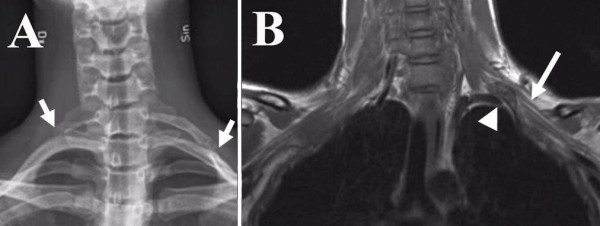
Case 2: (A) Frontal radiograph showing bilateral cervical ribs, shorter on the right side. The lateral end of the cervical ribs is marked with arrows. (B) MRI T1-weighted coronal image showing the cervical rib (arrow head, B) with its pseudoarthrotic bony formation that lifts up the brachial plexus (long arrow).
